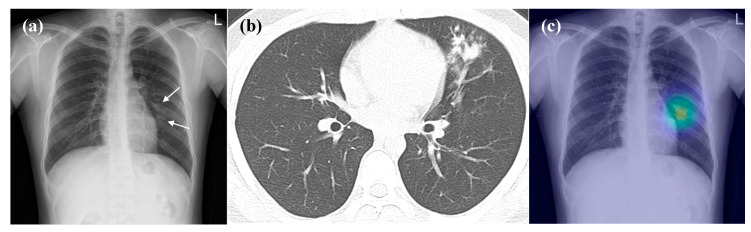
Representative case of the observer performance test. (a) The CR demonstrates patchy opacity in the left middle lung field (arrow), which was initially detected by only one of three observers. (b) The CT taken on the same day shows branching opacities and centrilobular nodules at the left upper lobe. (c) The DL algorithm correctly detected the lesion (probability score, 0.577). After assistance from the DL algorithm, all observers detected the lesion.
